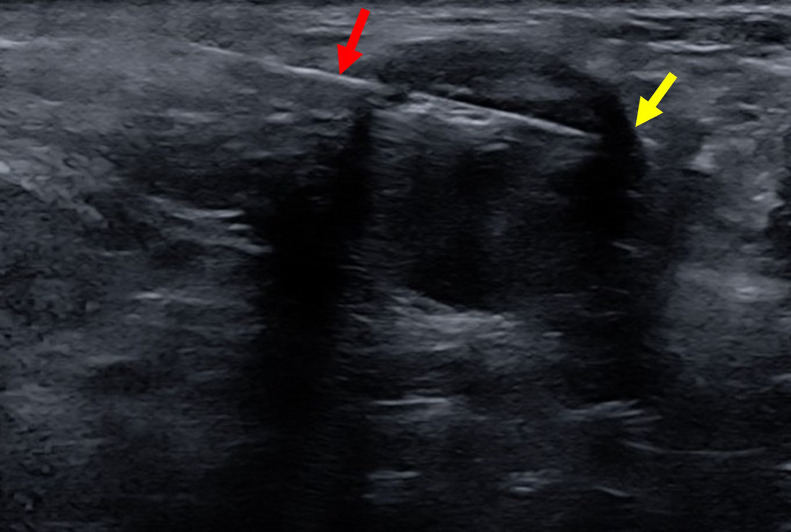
Transverse ultrasound image obtained during ultrasound-guided core needle biopsy of the right axillary lesion (yellow arrow). The 12-gauge biopsy guide (red arrow) was advanced into the lesion and 3 cores were obtained.
